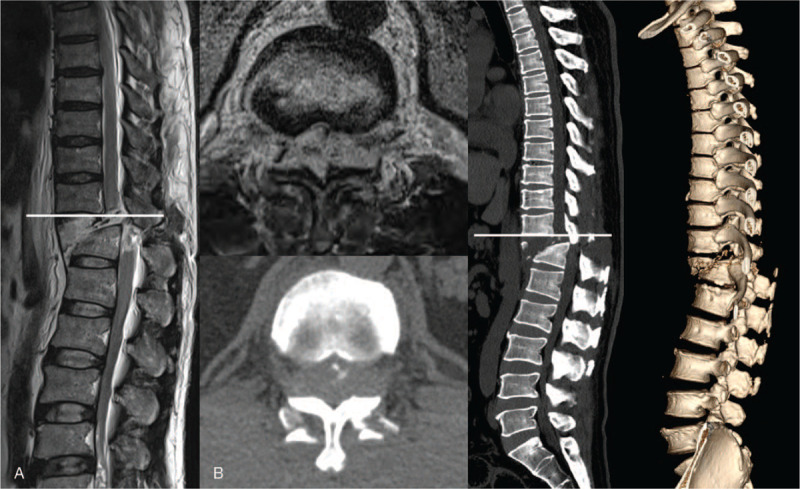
A. T2-weighted magnetic resonance imaging shows the dural defect. B. Three-dimensional computed tomography in the axial and reconstruction view shows fracture bone & fragment occupying the spinal canal.
